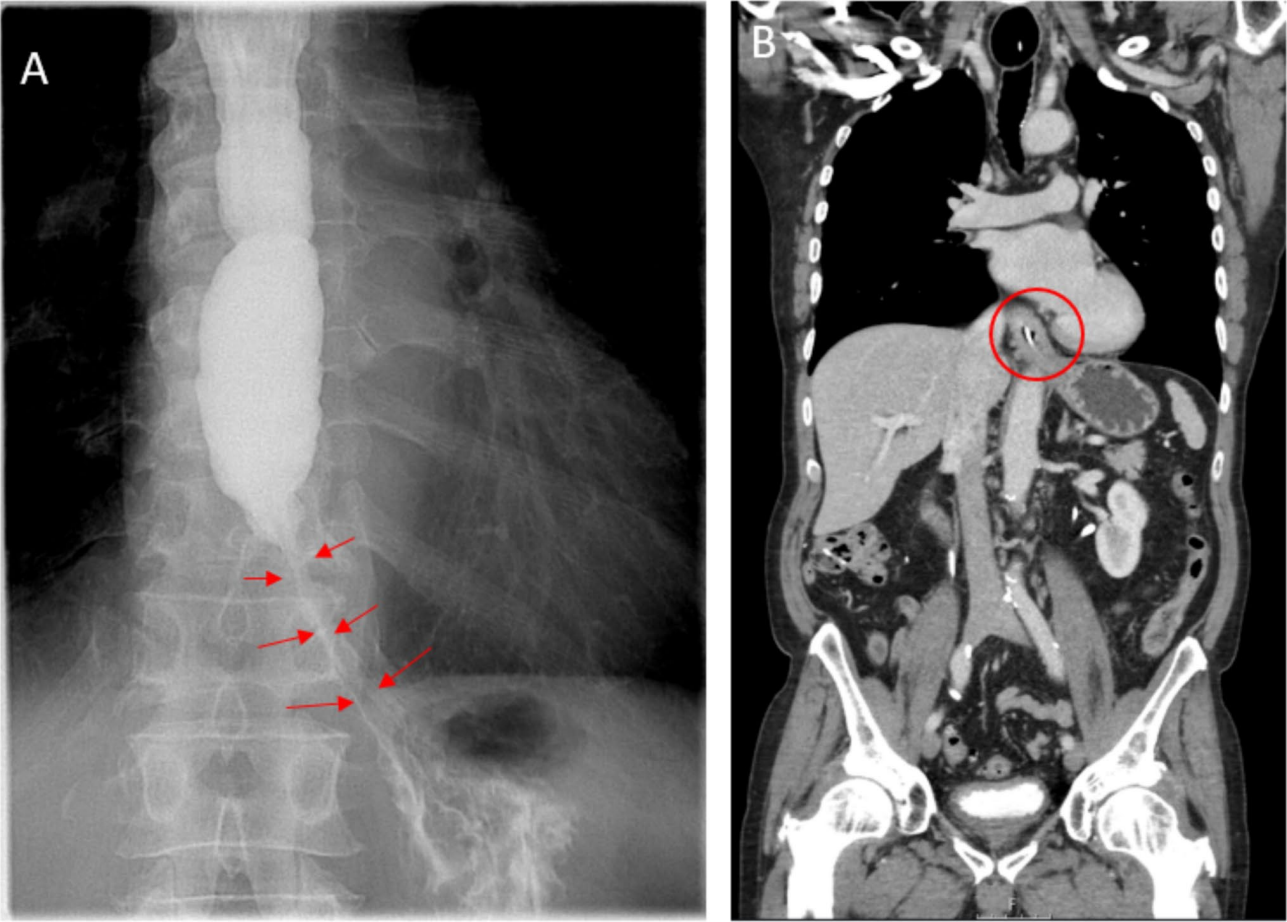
A The red arrows indicate a luminal narrowing in the distal esophagus on the barium swallow. B The area circled in red on the CT scan shows the distal esophagus with visualization of an inserted nasojejunal tube
